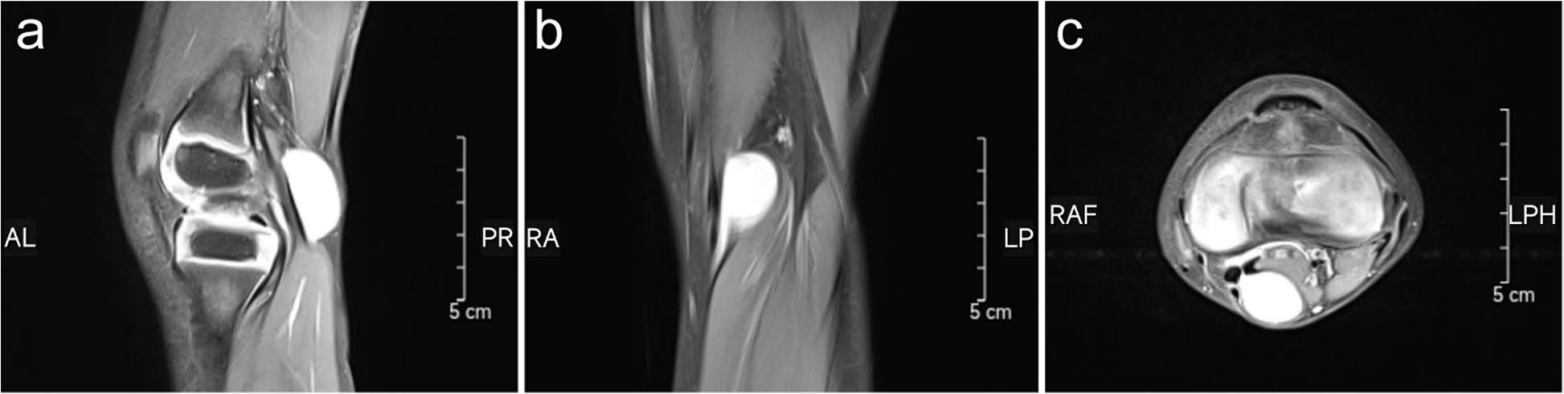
Pre-operative MR images of sagittal T2 (a), axial (b) and coronal view (c) demonstrate a popliteal cyst on the left knee
